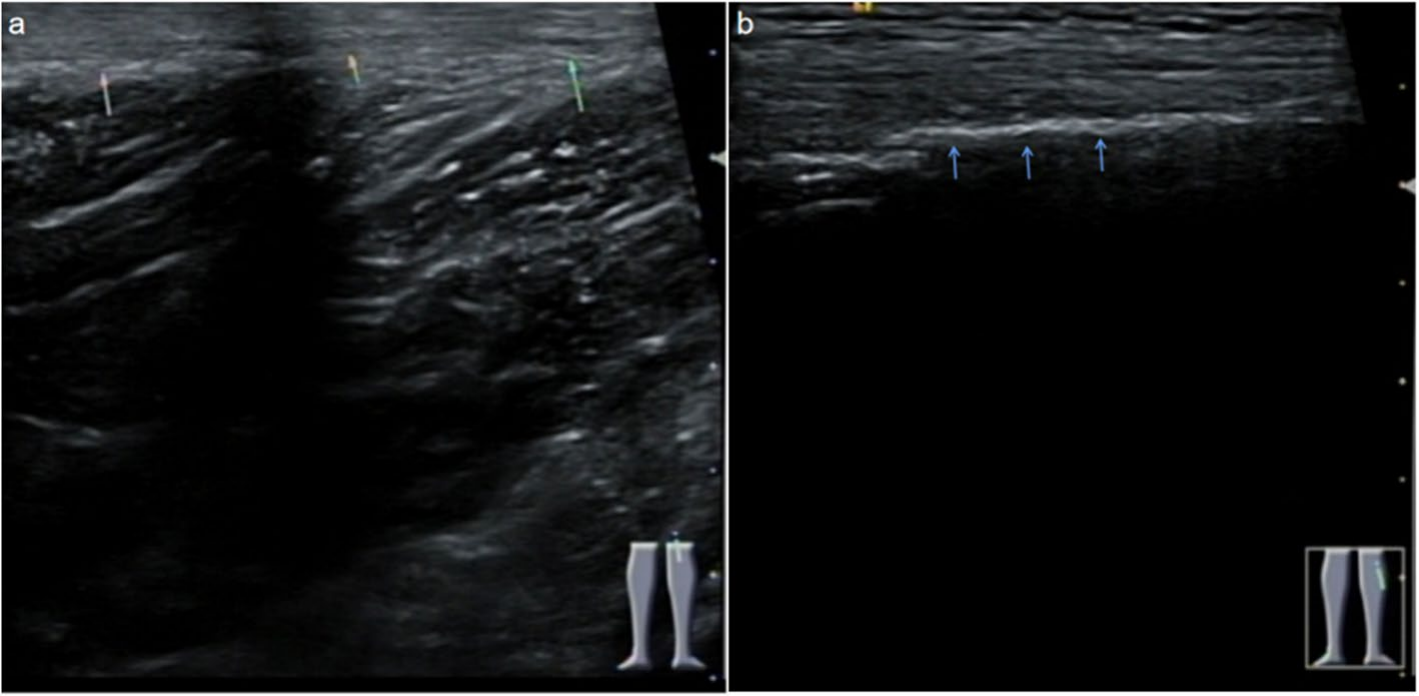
Ultrasound was used to explore the left semitendinosus tendon at the position of the popliteal fossa (a) and tibial attachment (b)
